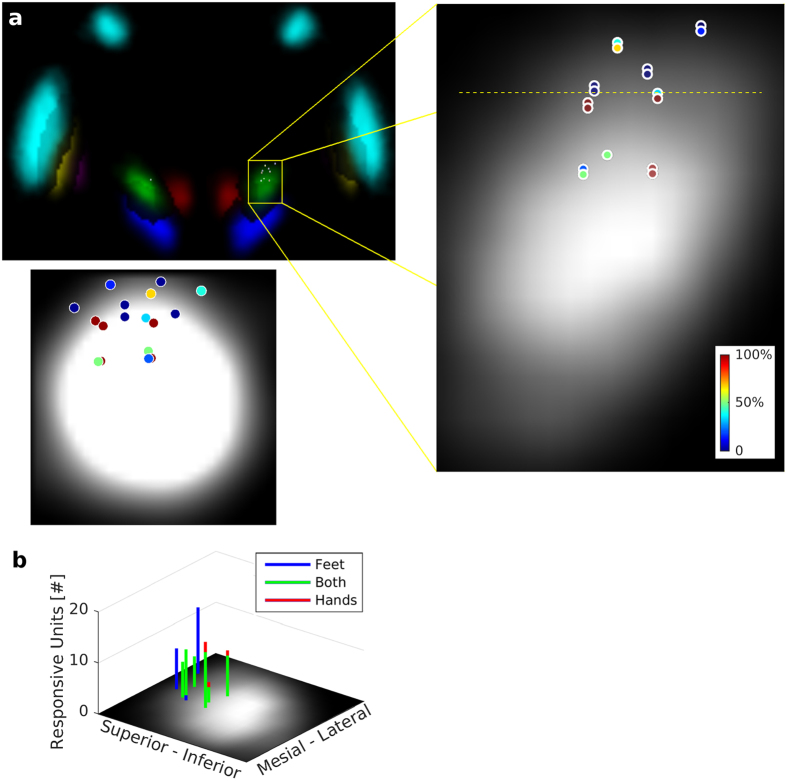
Localization of task-related neurons and somatotopy. (a) Recording sites marked on a coronal section of a 7T MRI subcortical probabilistic atlas^45^, 15 mm posterior to AC (STN: green; substantia nigra: blue; red nucleus: red; GPi: magenta; GPe: yellow; Striatum: cyan). In the expanded image, the gray background indicates the STN. Similarly, the bottom panel is a sagittal section 12 mm from the midline showing the STN as gray levels. In both sections, the location of each electrode is marked by a circle and colored according to the percentage of units recorded on that electrode which directly encode movement kinematics (see Results). Higher percentages of such units were recorded by electrodes inferior to the yellow dashed line, which is 3.6 mm below the AC-PC line. (b) Subthalamic somatotopic organization with overlap in the representation of feet and hand movements. The amount of units related to feet movements only (blue), hand movements only (red), and both hand movements and feet movements (green) is displayed as a stacked histogram plotted at recording locations. Units related solely to feet movements were found in the superior-mesial part only, whereas those related merely to hand movements lied in the more inferior-lateral part of the recording zone. The figure shows only 8 recording sites, because it depicts the results for the 9 patients implanted in the Right STN, and for one of these subjects this recording site did not yield any responsive units.
